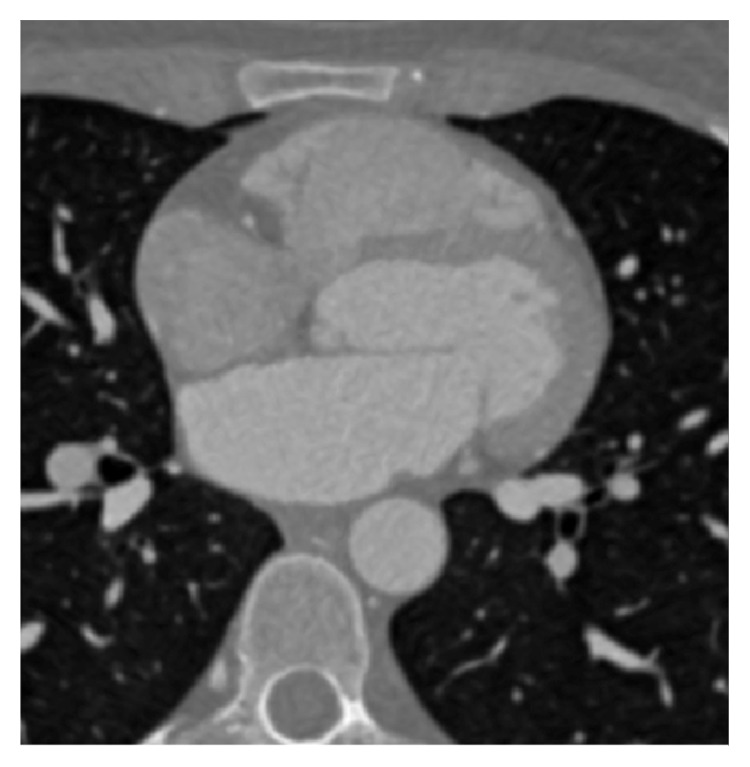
Input CT image slice of a heart, without additional contrast enhancement applied.
